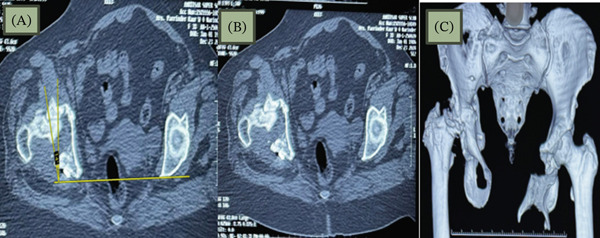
(A–C) CT demonstrating acetabular retroversion of 14°, vertical acetabular orientation, and external rotation of the right hemipelvis.
